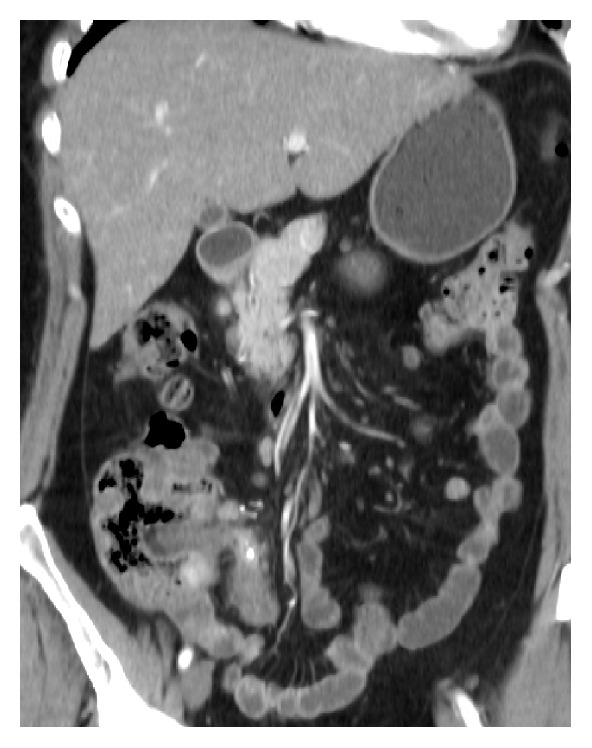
Appendicitis on abdominal CT, coronal plane.
